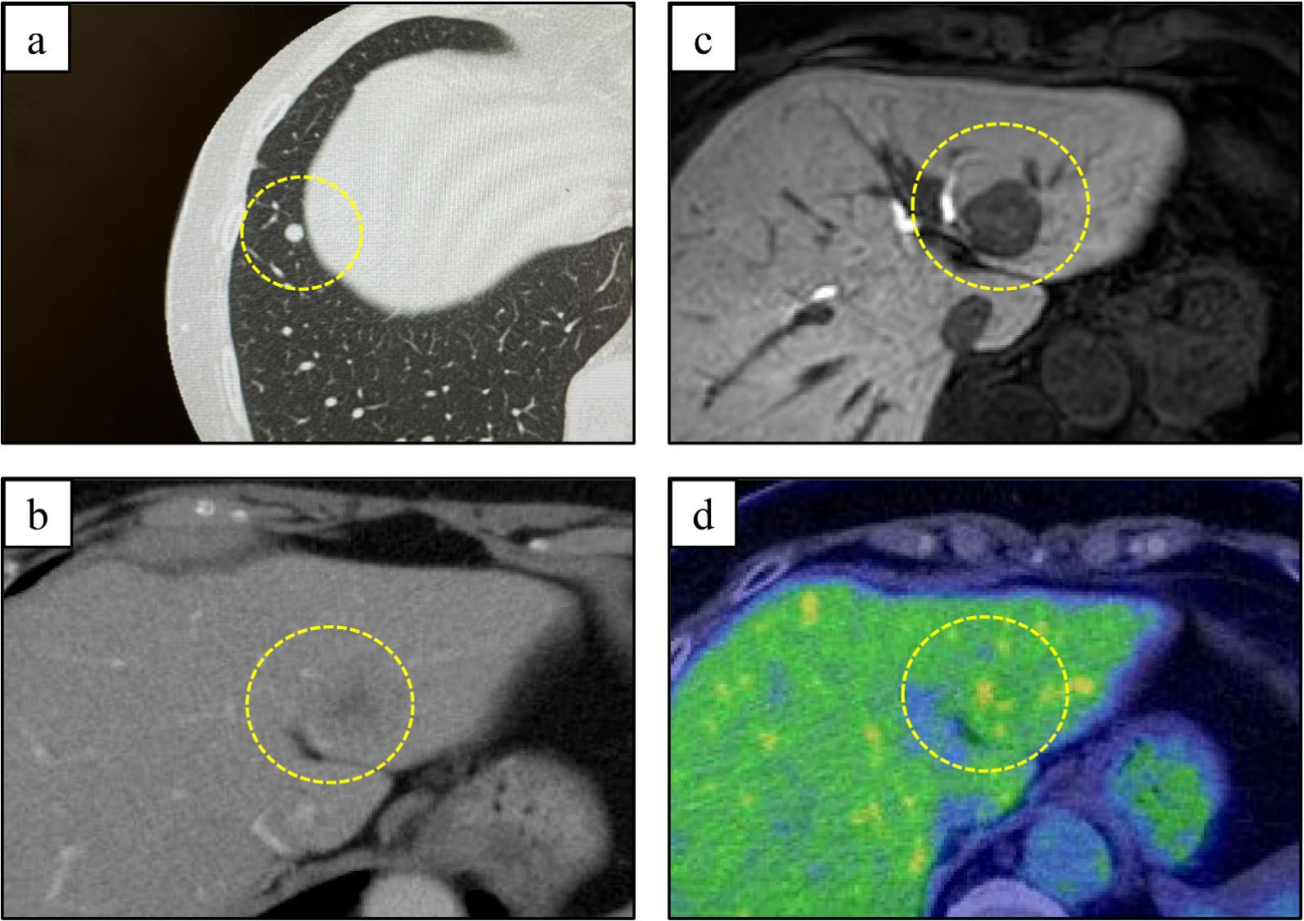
Imaging findings of the metastases. a Computed tomography image showing a single 5 mm mass (dashed line) in the lower lobe of the right lung. b Computed tomography image obtained 9 years after the first surgery showing a single 18 mm hypo-absorptive zone in liver segment II (dashed line). c Magnetic resonance imaging scan showed high intensities in the main lesion on T1-weighted imaging, suggesting melanin deposition (dashed line). d F-18-fluoro-2-deoxyglucose (FDG) positron emission tomography–computed tomography revealed mild FDG deposition in the main tumor with a maximum standardized uptake value of 3.76 (dashed line)
